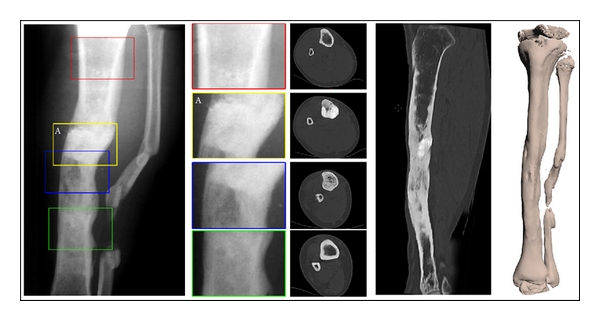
Bone lengthening in an area previously reconstructed with BCP. X-Ray and CT images of a tibia showing the zone reconstructed with BCP (A) and the new bone formed after osteotomy and bone lengthening, which was performed due to the shortening of the limb.
